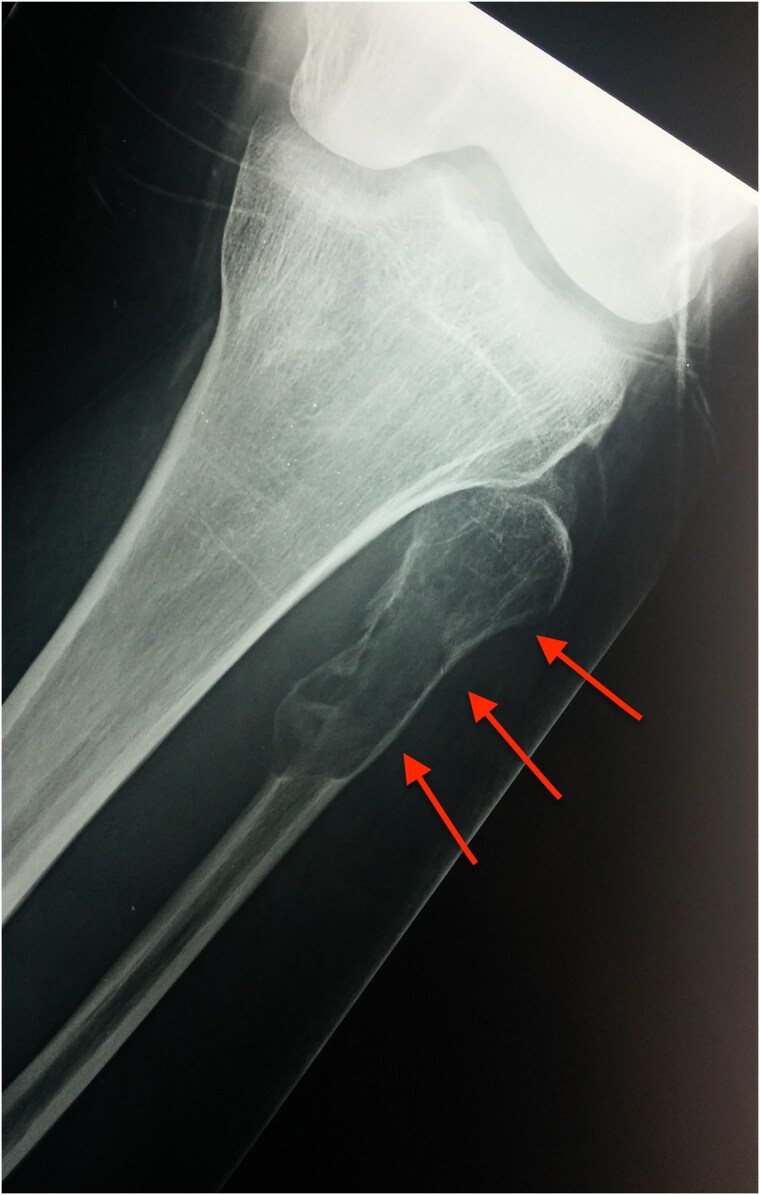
X-ray of the knee joint. Brown tumor of the fibula as a manifestation of primary hyperparathyroidism in a 52-year-old man.
